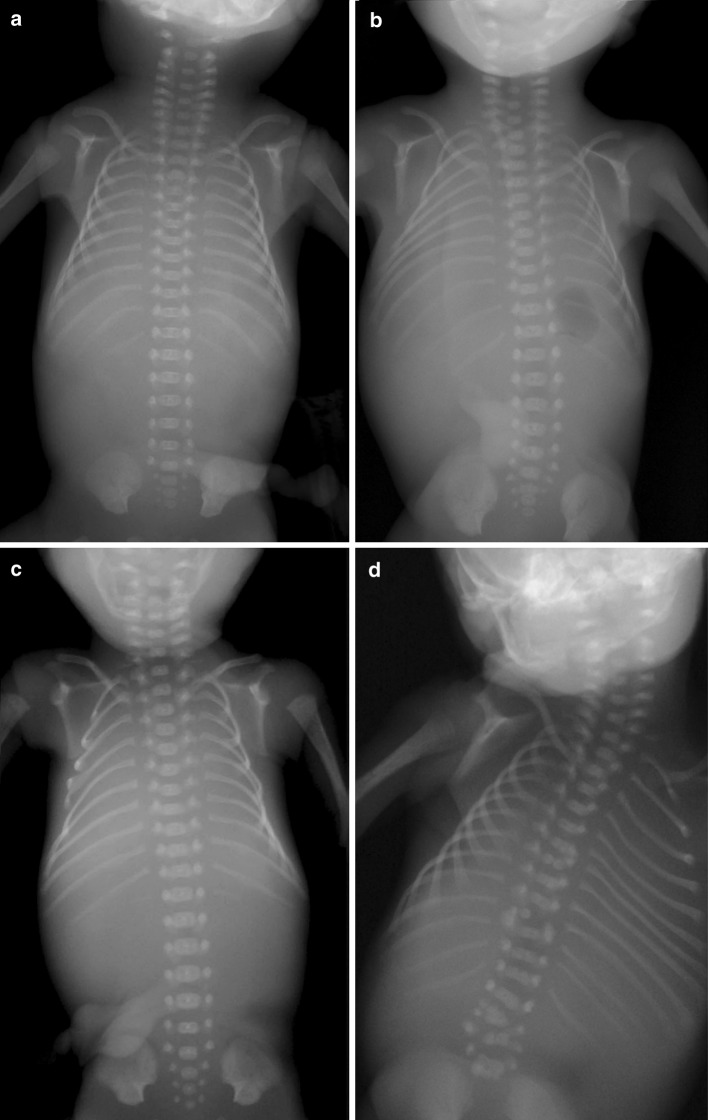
Radiographs of deceased fetuses with different vertebral patterns. a Regular pattern with 7 cervical, 12 thoracic and 5 lumbar vertebrae (pattern R). b Fetus with bilaterally rudimentary first thoracic ribs (Pattern CT, with cervico-thoracic boundary change). c Fetus with unilaterally a cervical rib and bilaterally absent twelfth thoracic ribs (Pattern CT_TL, with changes of the cervico-thoracic and thoraco-lumbar boundary). d Fetus with bilaterally cervical ribs and full-sized lumbar ribs (Pattern CT_TL, with changes of the cervico-thoracic and thoraco-lumbar boundary). The vertebrae and ribs are highly irregular with hemivertebrae and fused ribs, these represent segmentation defects
